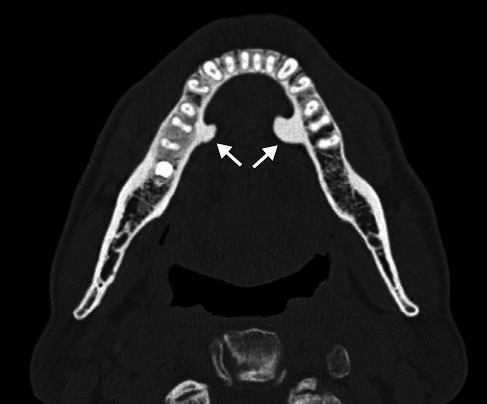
Mandibular torus in a 68-year-old male patient. Axial CT image
